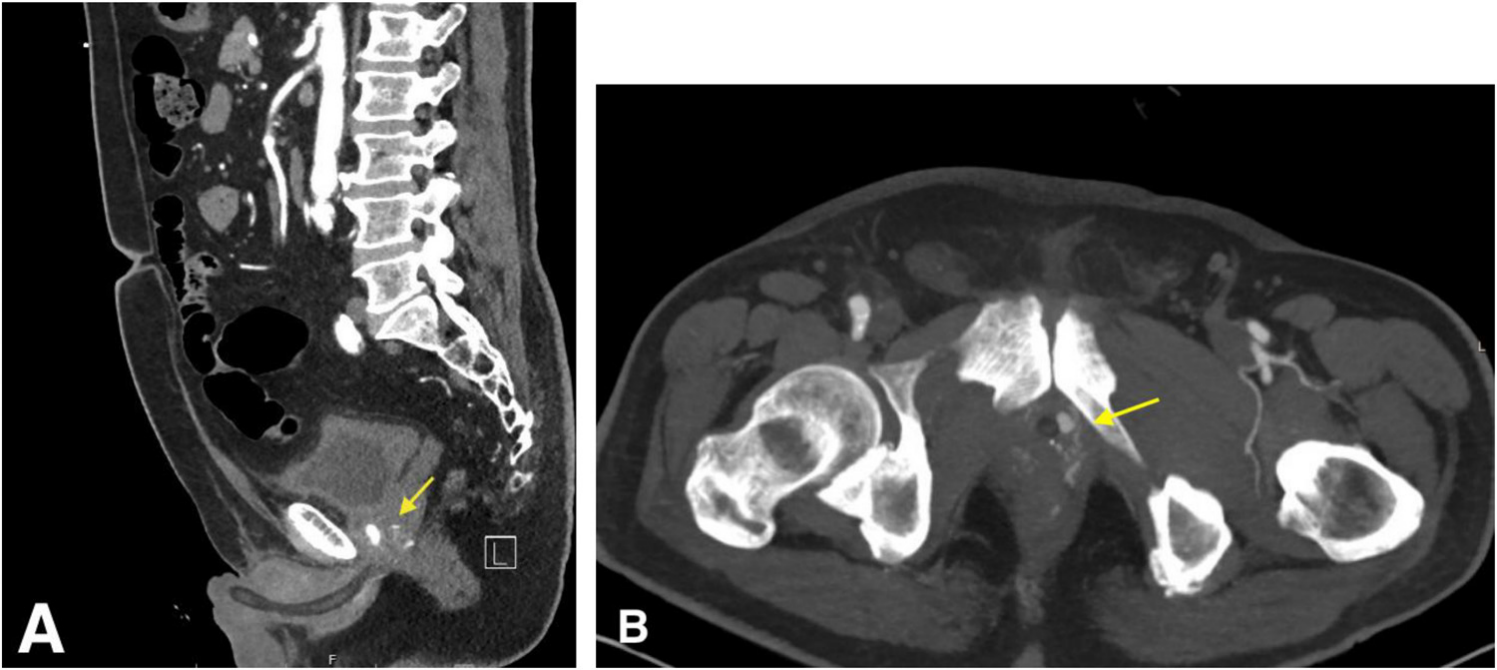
CTA of the pelvis demonstrates a left prostatic artery pseudoaneurysm. (A) Sagittal contrast-enhanced CT angiography demonstrates a well-defined, contrast-opacified pseudoaneurysm (arrow) at the left prostatic apex, along the lateral margin of the prostatic urethra. (B) Axial CT angiography again shows the pseudoaneurysm (arrow) adjacent to the Foley catheter, measuring 1.1 × 0.6 cm, with increased arterial enhancement consistent with prostatic arterial supply.
